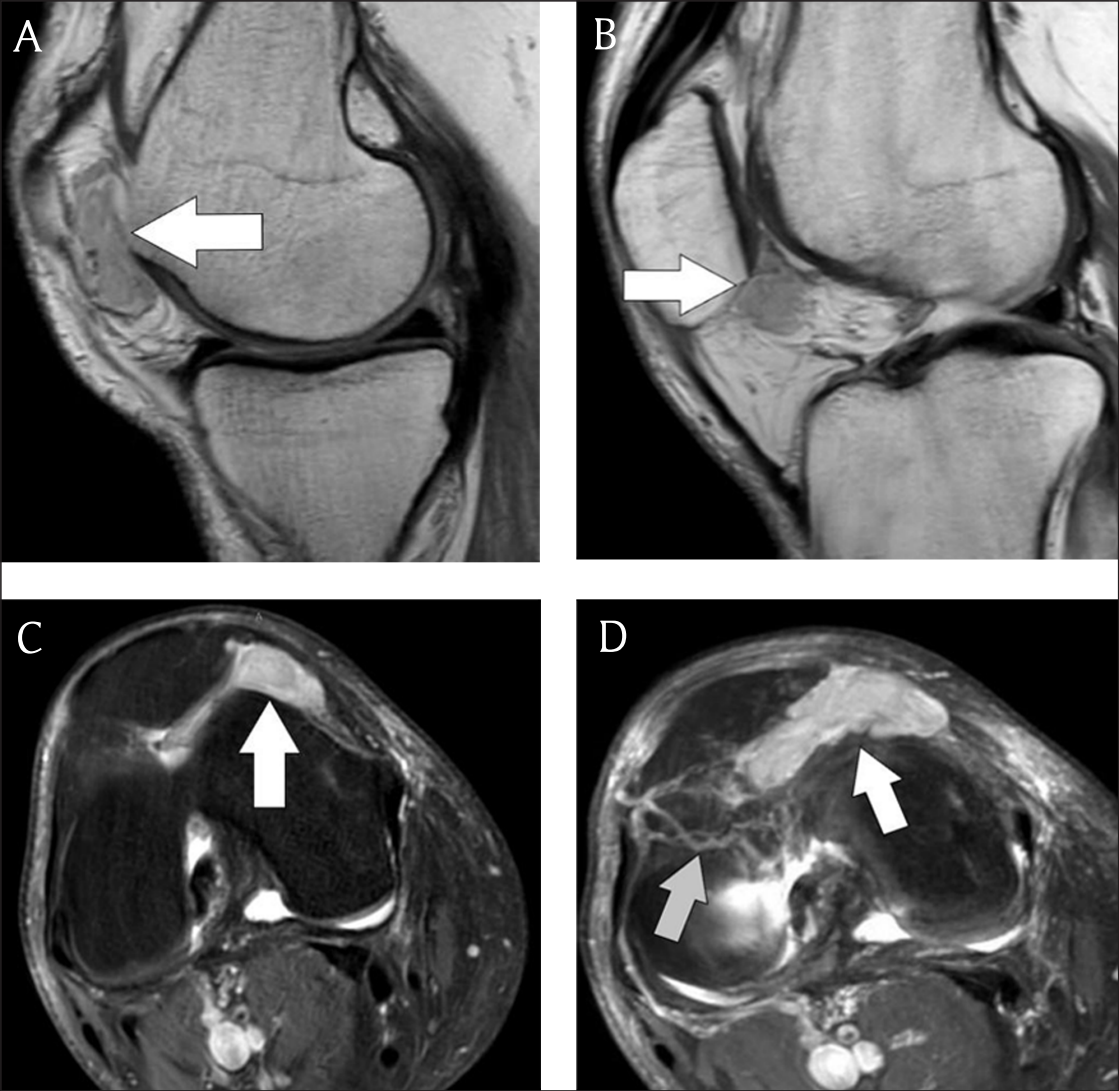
MRI imaging of the right knee. A and B. Sagittal T1-WI (2 adjacent slices) shows a well circumscribed solid mass (*white arrow*) in the infrapatellar fat pad that is isointense to muscle. The lesion shows a close relationship with the underlying synovium of the joint space. C. Axial fat-suppressed proton density T2-WI shows a hyperintense mass (*white arrow*) extending into the medial part of the patellofemoral joint that is limited by the medial collateral ligament. D. Axial fat-suppressed T1-WI after gadolinium contrast administration. Note vivid homogeneous contrast enhancement of the mass (*white arrow*) and the presence of tortuous feeding vessels (*grey arrow*).
